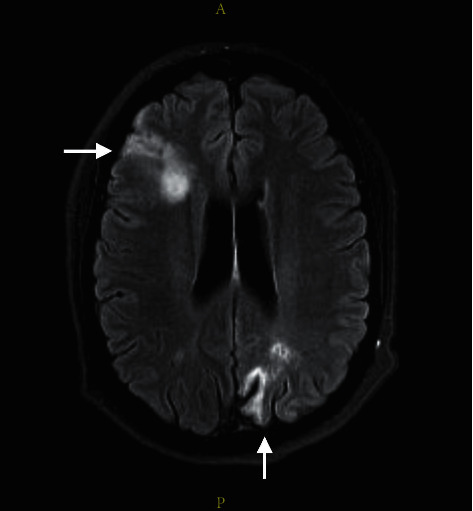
Contrast MRI brain (T2 FLAIR) showing right frontal subacute and left occipital chronic infarctions.
